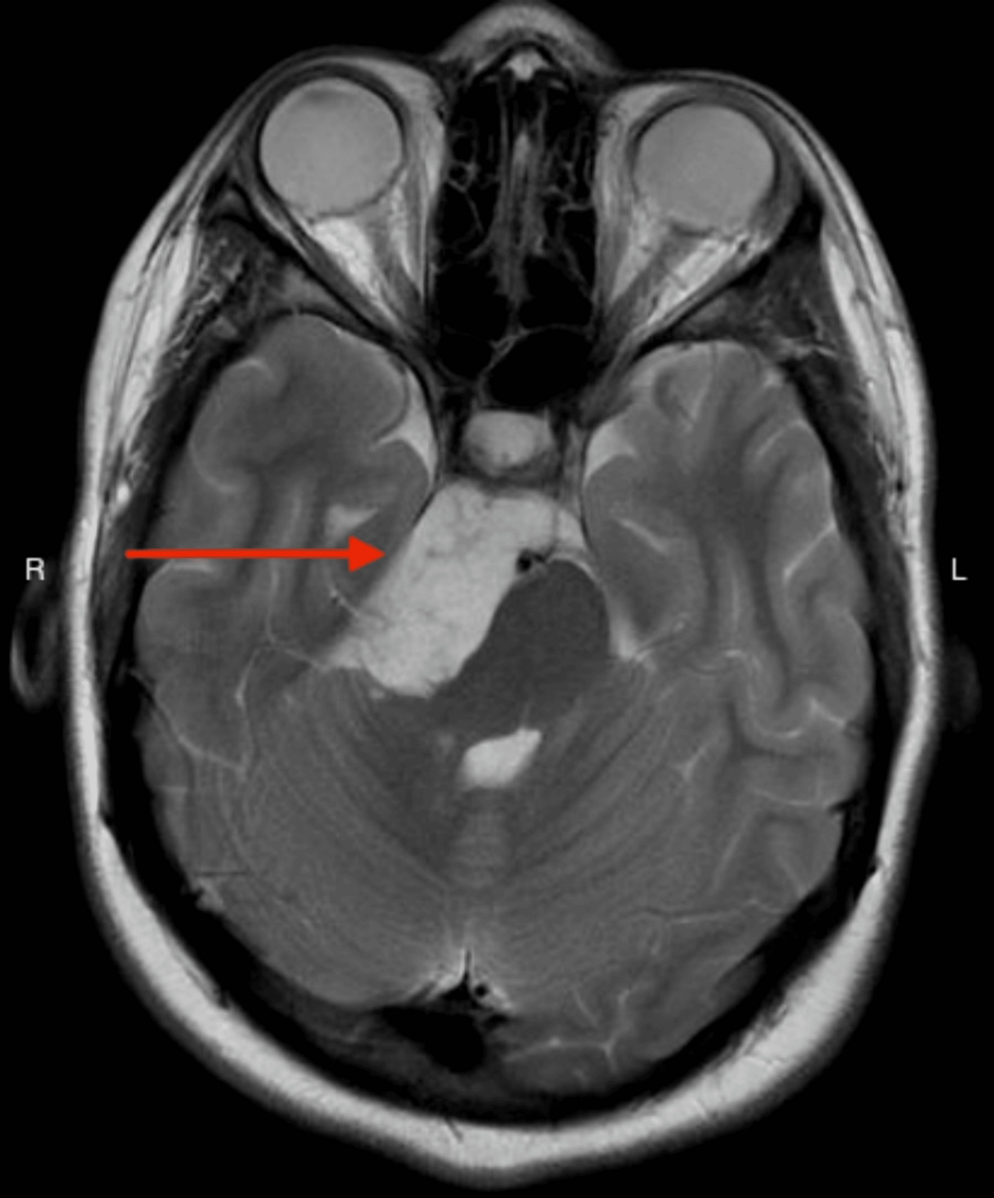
MRI of the brain Axial T2-weighted MRI image showing hyperintense space-occupying lesion (arrow). MRI: magnetic resonance imaging.
